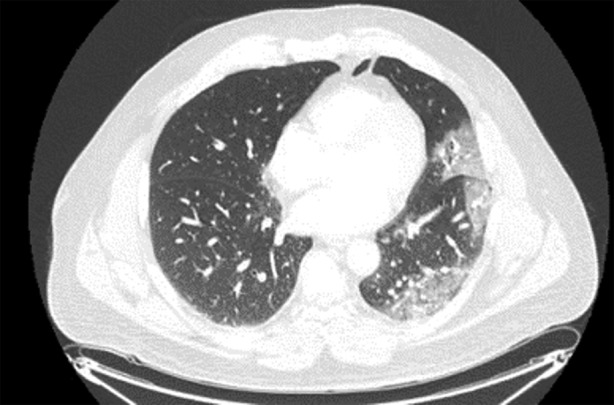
Chest non‐contrast axial computed tomography (CT) scan, note ground‐glass opacity of the left lung.
